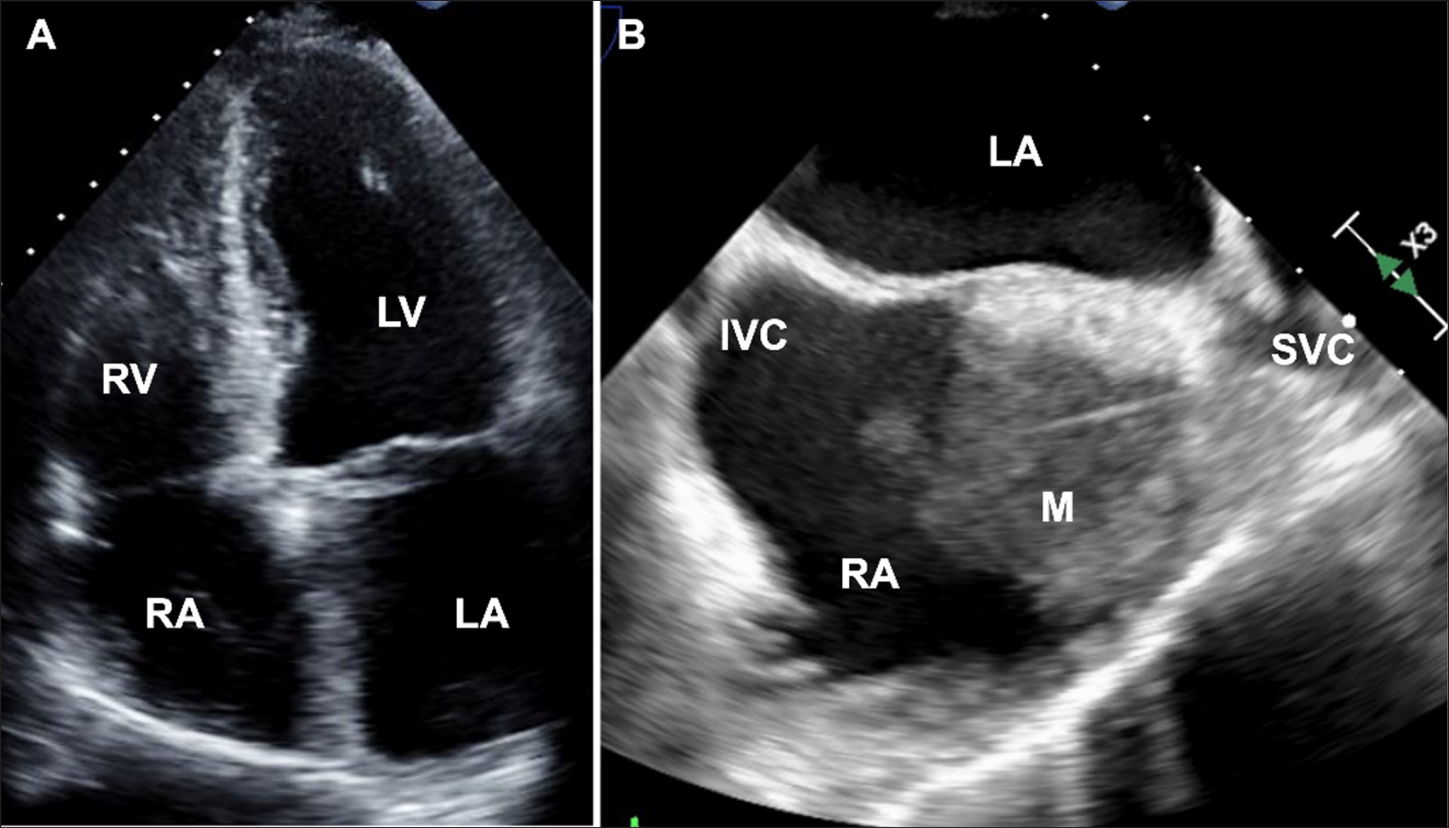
(A) Apical four-chamber view on transthoracic echocardiogram did not show any evidence of mass or thrombus in the RA. (B) Mid-esophageal bicaval view on transesophageal echocardiogram shows the mass extending from the superior vena cava into the RA along the device lead. RA: right atrium; LA: left atrium; IVC: inferior vena cava; M: mass; SVC: superior vena cava
